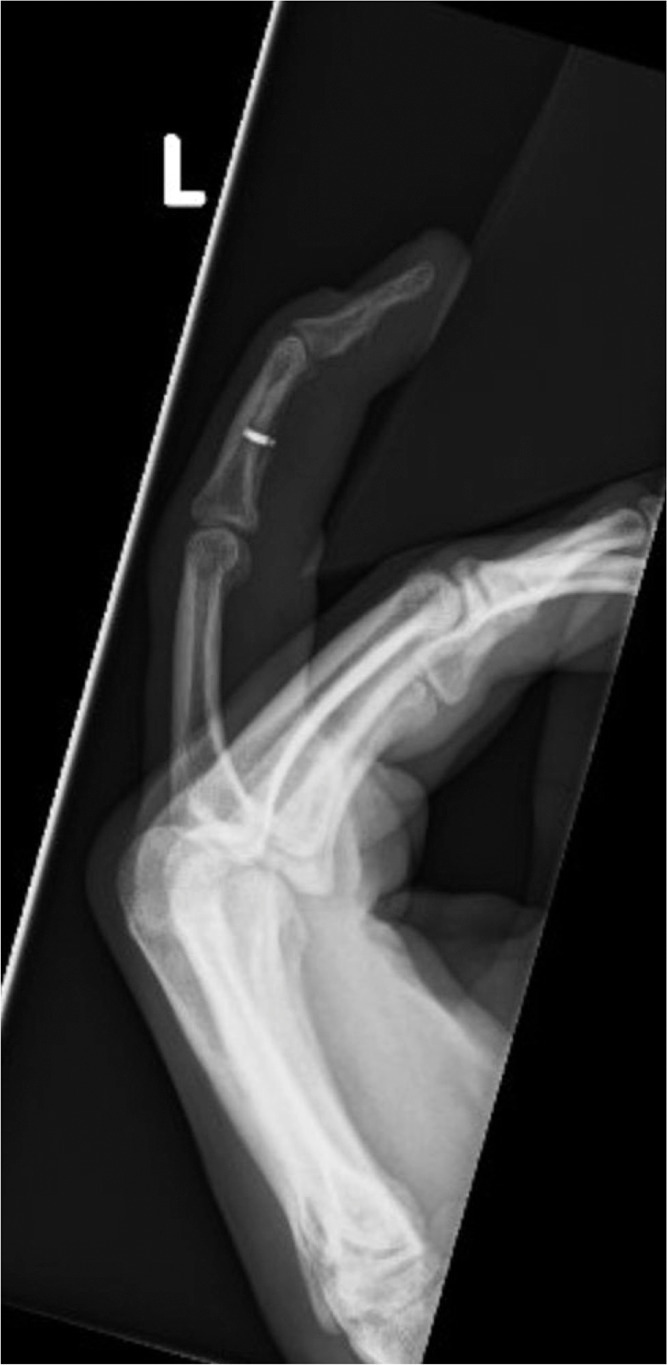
Lateral X-Ray of right hand (Case 2: Post-op).
